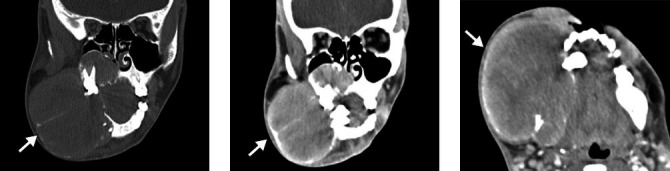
Brown tumor in a 21-year-old male patient. CT scans showing an
